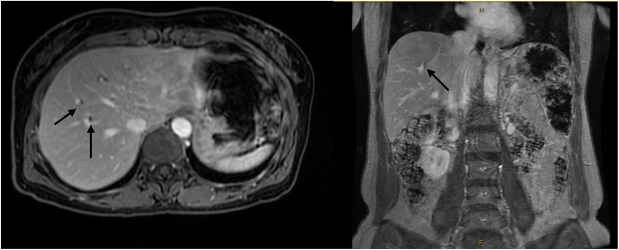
Magnetic resonance imaging liver/MRCP demonstrating subtle intrahepatic biliary dilatation (arrows), most pronounced in segment 8.
